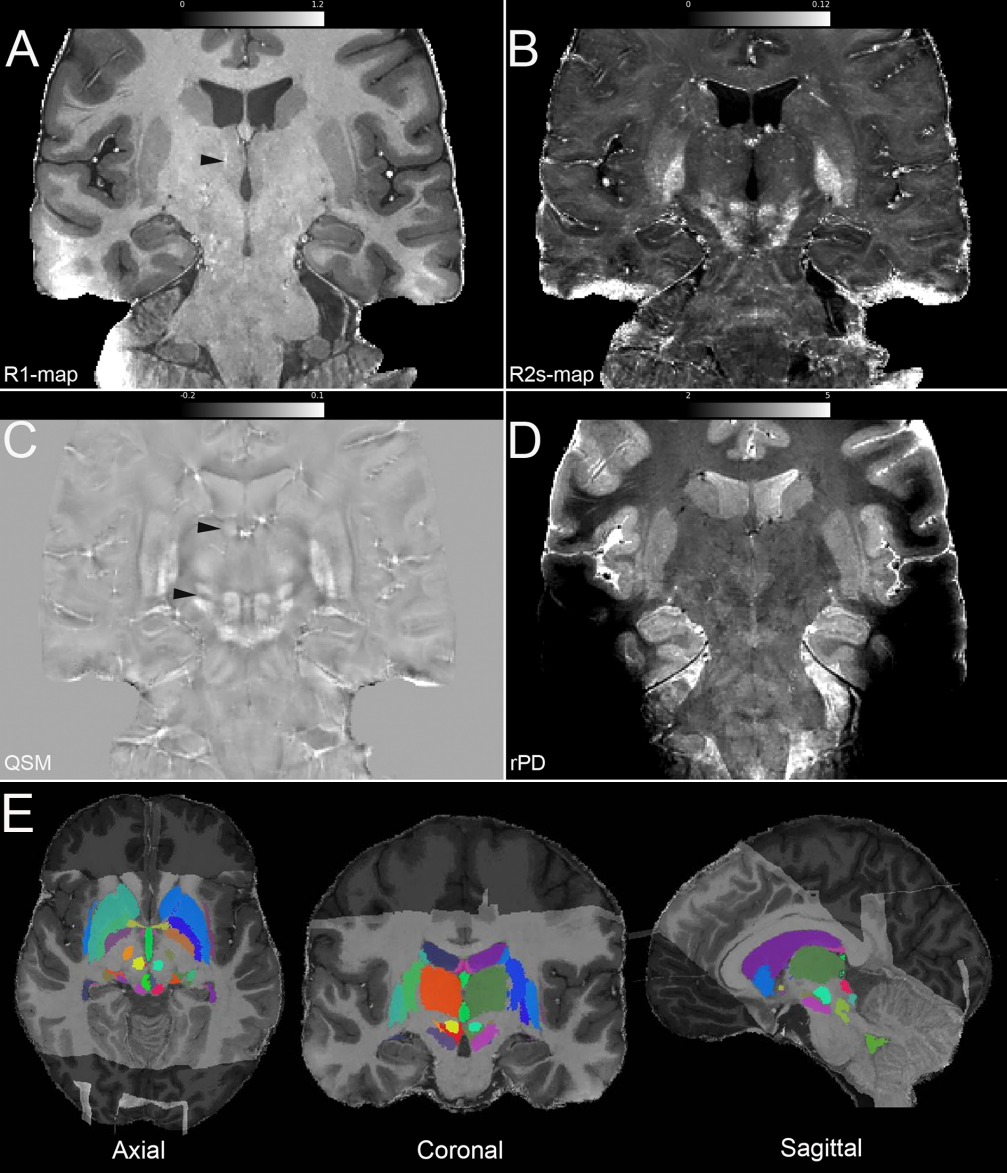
Example of the 0.5 mm isotropic 7T MRI data with partial brain coverage. The arrowhead in the R1-map (A) indicates the visibility of the thalamic lamina. (B) shows the R2*-map. In the QSM (C), you can distinguish the anteroprincipal nucleus of the thalamus as well as the border between the substantia nigra and the subthalamic nucleus can be distinguished (arrowheads). (D) shows the relative Proton Density (E) shows the combination of the slab with the whole-brain data and the delineations created with MASSP2.0.
